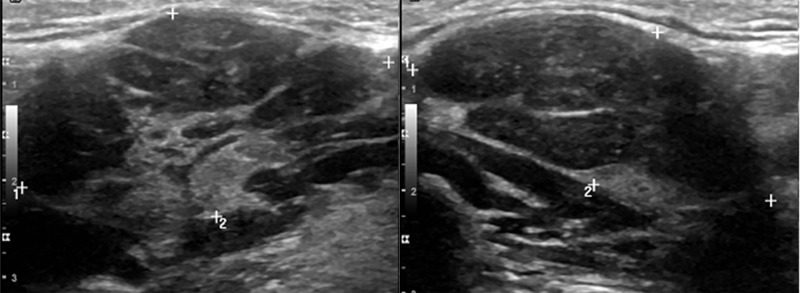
Ultrasound of the neck reveals enlargement of the right submandibular gland measuring 4.0 x 2.2 cm and the left submandibular gland measuring 2.7 x 1.6 cm.
